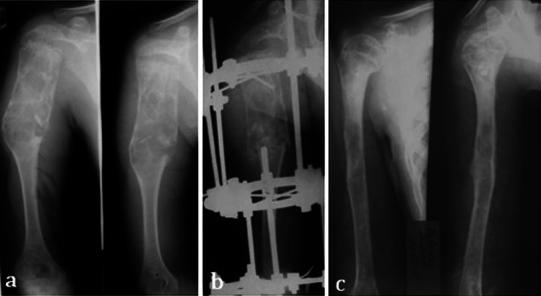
a X-ray of aneurysmal bone cyst (ABC) of right humerus in a 13-year-old boy. b Application of external fixator and open osteotomy and coaptation.c Capanna grade 1, completely healed, after 15 months
